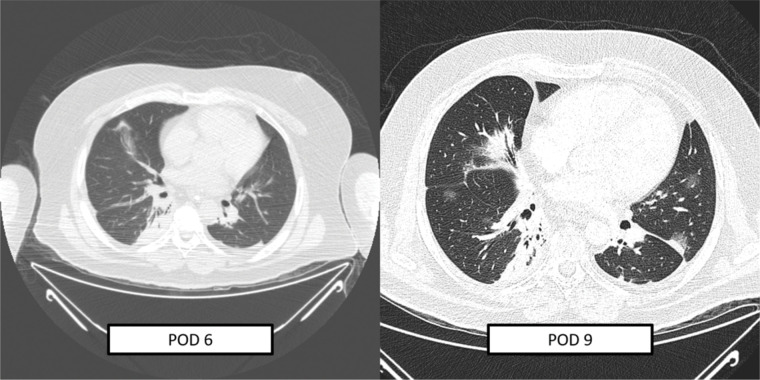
Evolutionary changes in thoracic computed tomography (CT) scans from the sixth to the ninth postoperative days (PODs).
